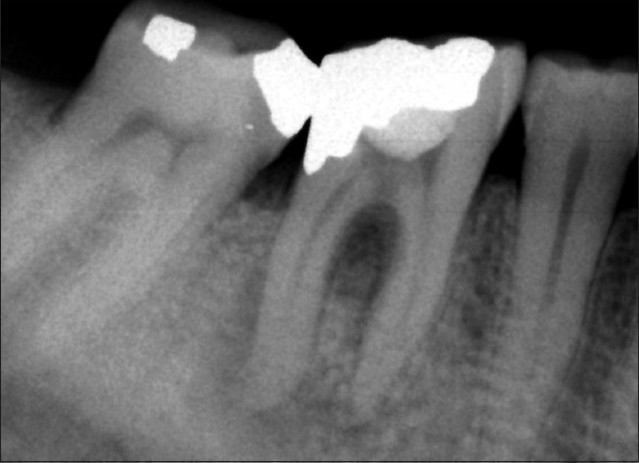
Initial periapical image of left first mandibular molar showing furcal perforation and apical lesions
